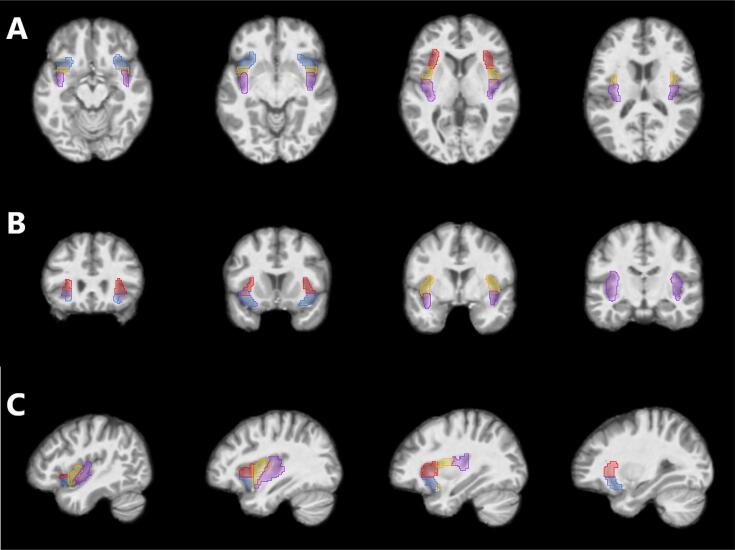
Regions of interest defining the different insular subregions on MRI T1 brain images. A- Axial planes in inferior to superior direction. B- Coronal planes in anterior to posterior direction. C- Sagittal planes of the right hemisphere in lateral to medial direction. Red – dorsal anterior insula; Blue – ventral anterior insula; Yellow – middle insula; Purple – posterior insula. (For interpretation of the references to colour in this figure legend, the reader is referred to the web version of this article.)
